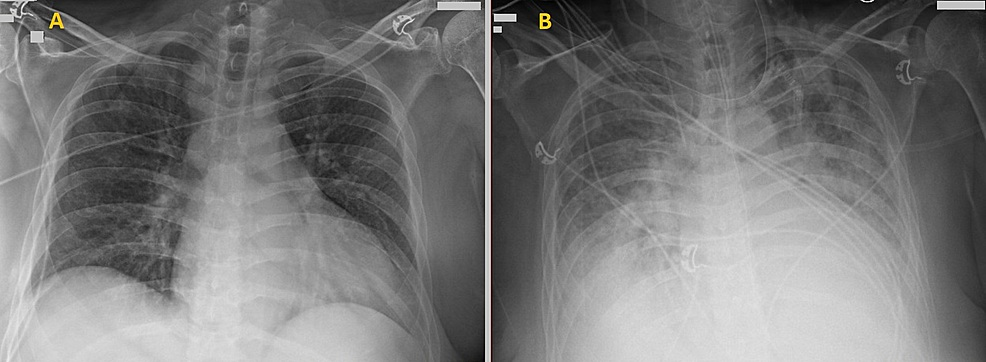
Chest X-ray on admission and two days later 2A: Chest X-ray on admission showing bibasilar atelectasis and superior mediastinal prominence concerning for adenopathy or mass. Two days later (Figure 2B), new moderate pulmonary edema developed.
